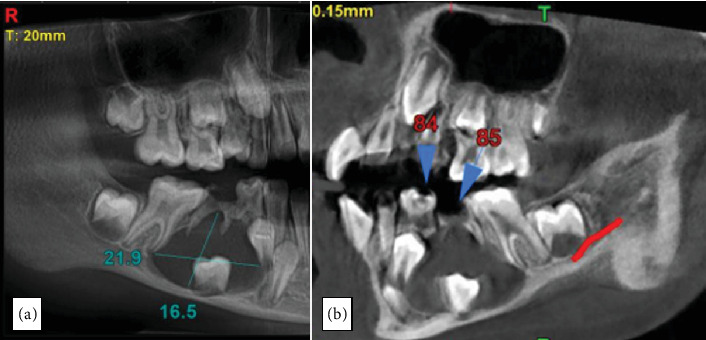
CBCT showing (a) extent of the lesion and (b) its relation with mandibular canal.
